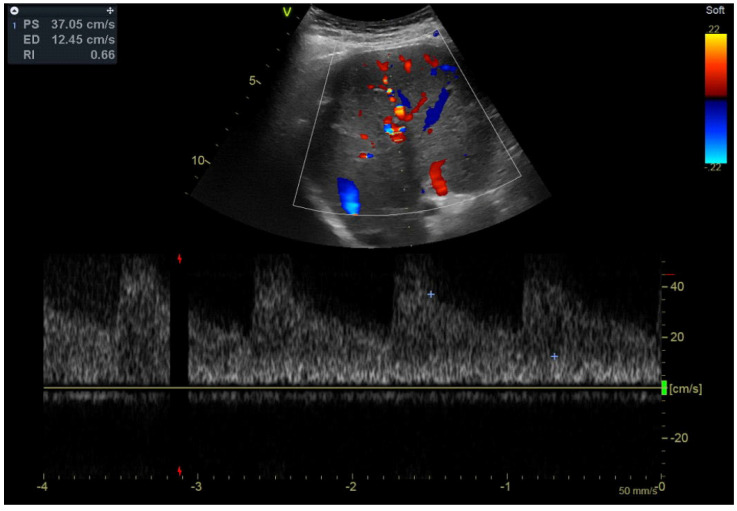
Doppler ultrasound of the hepatic artery with an increased compensatory flow. *Source*: AG.
